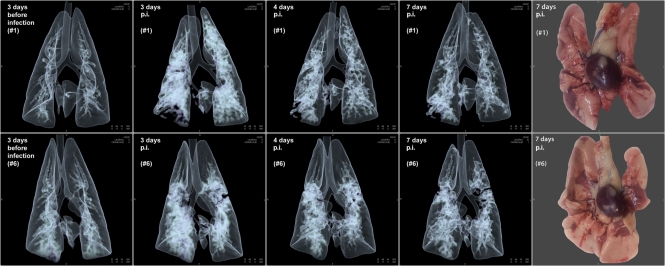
Two rows of four consecutive 3D lung CT images of ferrets #1 and #6 recorded *in vivo* under BSL-3^+^ compared with their appearance at necropsy on the far right. At day 3 before infection, the lungs showed the clear aerated baseline condition, at day 3 p.i. with the new pandemic H1N1 influenza virus marked almost diffuse ground-glass opacities are present that show a gradual reduction towards 7 days p.i. The two photographs taken at necropsy on 7 days p.i. depict the ventral aspect of the lungs, within the centre the hearts still attached to the pulmonary hilus. Both lungs show multifocal reddish consolidated areas of acute inflammation that essentially match with the opacities on the CT images taken just before necropsy; non-affected aerated lung tissue is light pink in colour.
